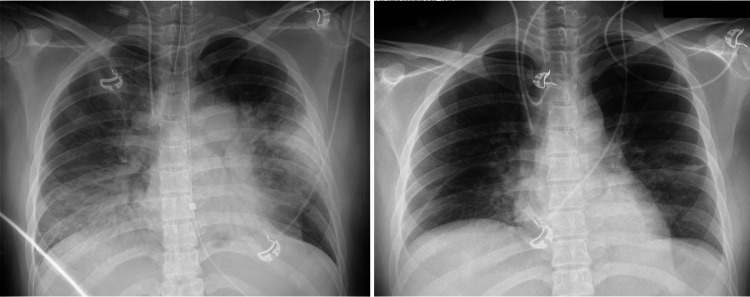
Chest radiography on day 1 and day 7 Chest radiography on day 1 (left side) revealed bilateral lung infiltration; however, on day 7 (right side), there was a significant improvement in the infiltration.
